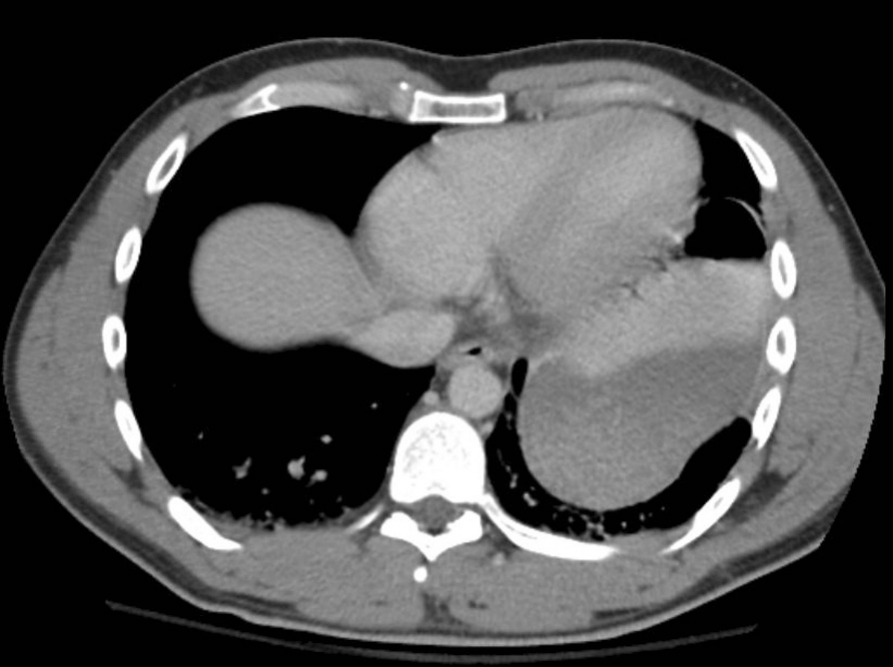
Computed tomography scan image showing the herniation of the stomach into the chest.
